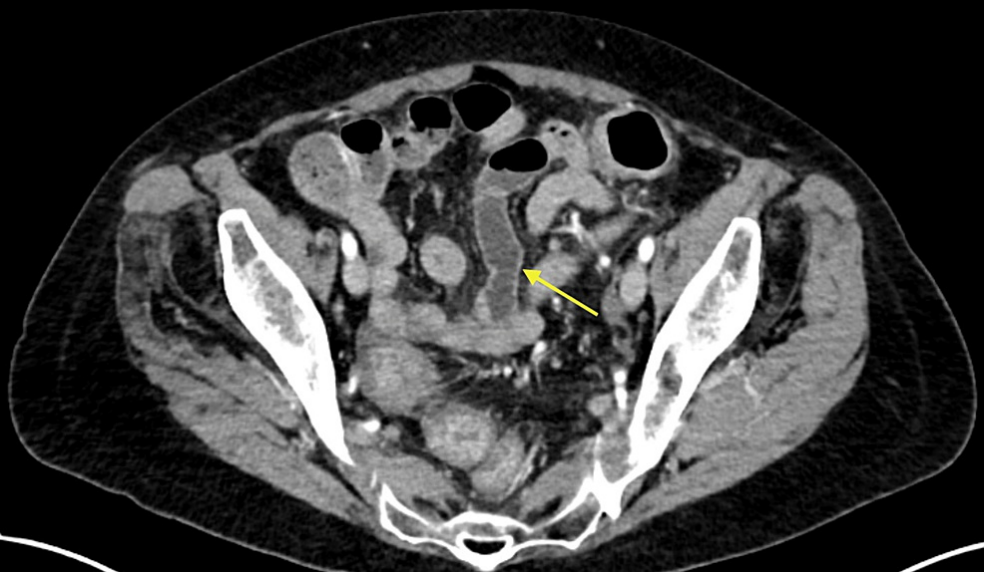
CT scan of the abdomen and pelvis with contrast depicting pancolitis with wall thickening CT: computed tomography
